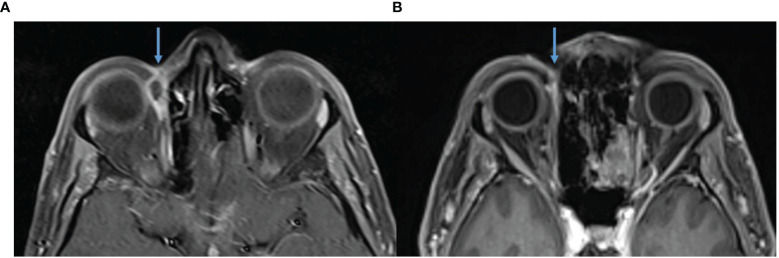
(A) Baseline, left, MRI post contrast T1 Fat Sat demonstrating 18 × 9 mm right medial extraconal orbital peripherally enhancing mass. (B) MRI post contrast T1 Fat Sat, post five cycles of pembrolizumab 200 mg/3-weekly, no mass like enhancement.
